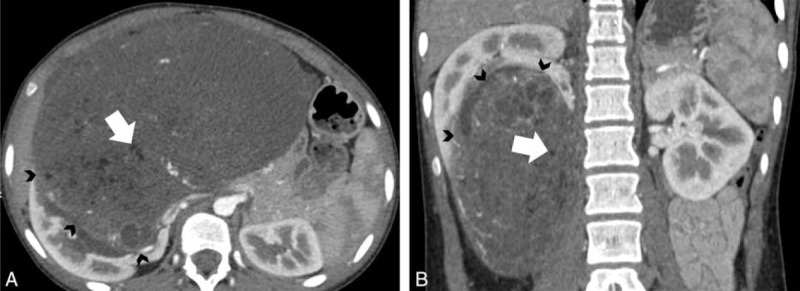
A 14-year-old woman with angiomyolipoma. A, Axial and B, coronal contrast-enhanced computed tomography images show fatty (white arrow) mass arising from right kidney with enlargement of renal sinus (black arrowheads).
